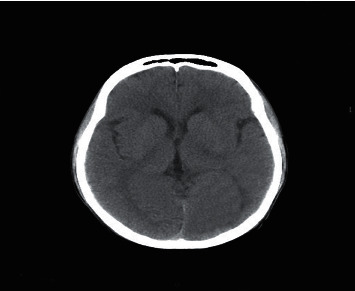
Normal brain on CT-scan.
